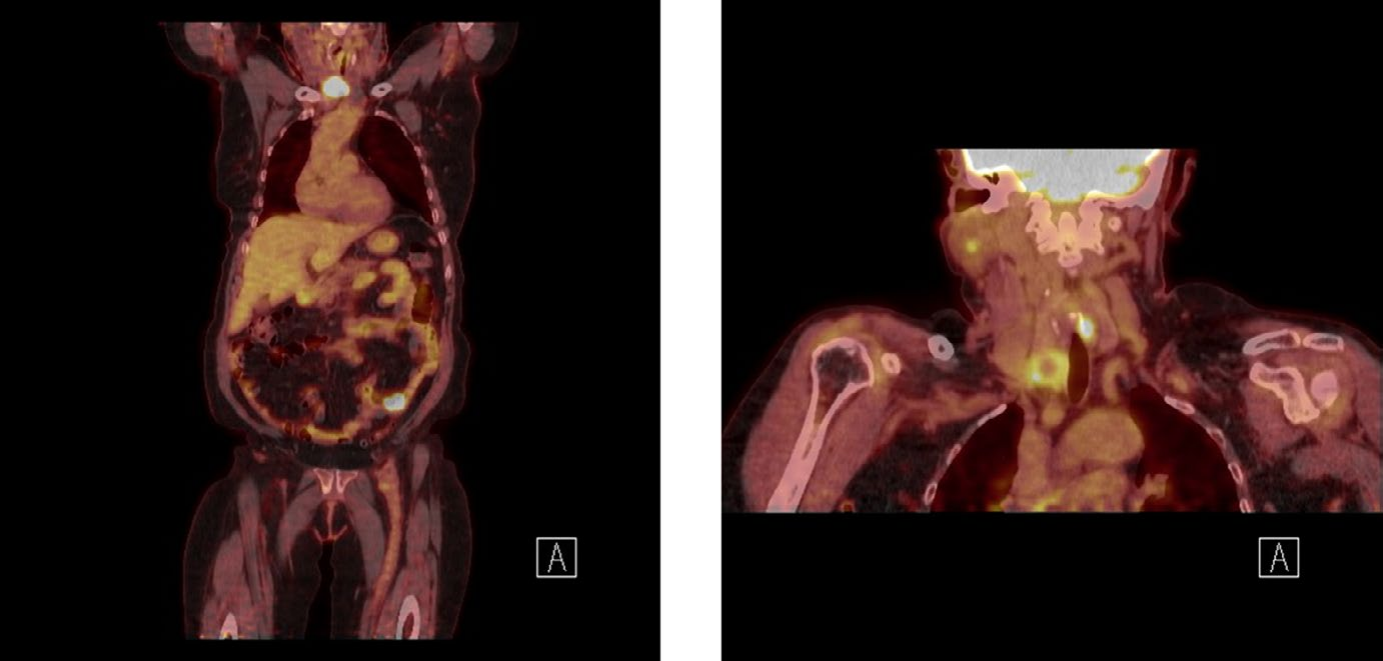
PET‐CT with distant disease within the small bowel (A) and lung (B) as well as inguinal lymphadenopathy (A)
